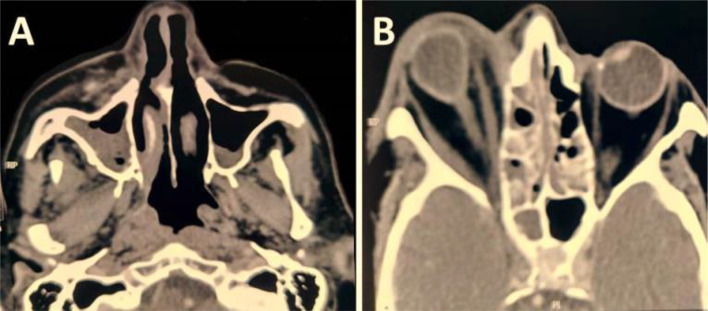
CT scan findings in a 68-year-old patient with COVID-19-related ROCM. A- Axial view of the nose and paranasal sinuses showing features of right maxillary sinusitis. B- Axial view showing right-sided proptosis, ethmoidal sinusitis, thickening of medial and lateral recti, and fat stranding in both intra- and extra-conal parts and the orbital apex.
